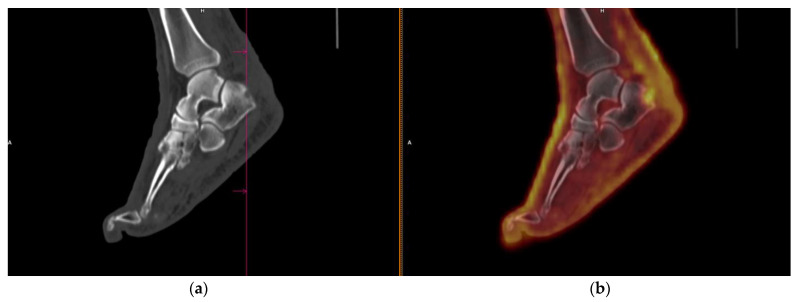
Classic KS. FDG PET/CT identifying hypermetabolic (SUV_max_ = 4.7) involvement of calcaneum contiguous to cutaneous and subcutaneous lesions. (a) Sagittal CT slice; (b) Sagittal-fused PET/CT slice.
